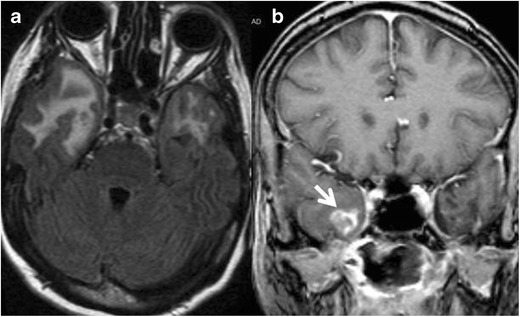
A 42-year-old female with a history of radiation for nasopharyngeal carcinoma. Axial FLAIR image (a) shows vasogenic edema in the anterior aspect of the temporal lobes bilaterally, right greater than left. Coronal contrasted T1 weighted image (b) shows enhancement on the right (*arrow*). On biopsy, the diagnosis of radiation necrosis was confirmed
